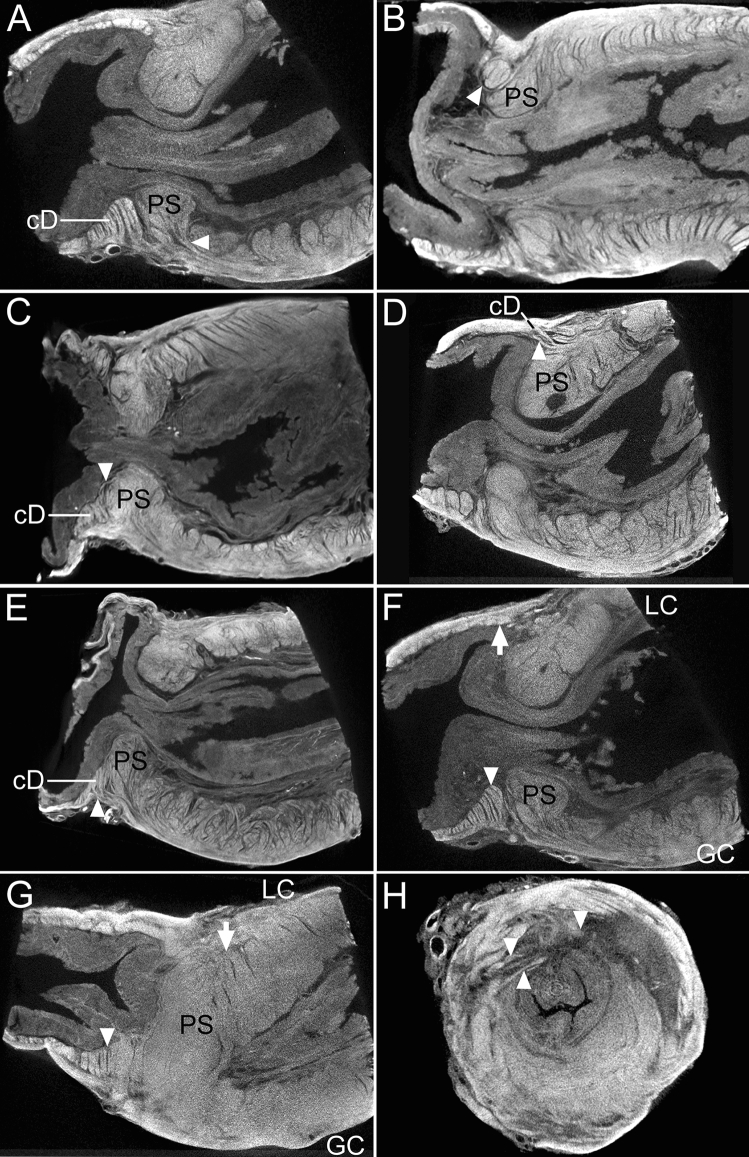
Microcomputed tomography images of the pyloric sphincter (PS). (A) Some outer longitudinal fibers (arrowhead) entered the PS in the longitudinal plane. (B) Some longitudinal fibers (arrowhead) entering the PS were connected to the aboral circular PS bundles in the longitudinal plane. (C) Some inner longitudinal bundles (arrowhead) were divided at the internal surface of the intermediate PS wall to connect to the circular bundles of the duodenum (cD) (arrowhead), adjacent to the PS in the longitudinal plane. (D) Some inner longitudinal bundles (arrowhead) that divided at the internal surface of the aboral end of the PS wall were connected to the cD adjacent to the PS in the longitudinal plane. (E) Some outer longitudinal bundles (arrowhead) were connected to the cD adjacent to the PS. (F) The cD (arrowhead) adjacent to the PS on the greater curvature (GC) side were thicker than those (arrow) on the lesser curvature (LC) side in the longitudinal plane. (G) In the longitudinal plane, the thick PS layer (arrow) on the LC side, and the thick duodenum muscular layer (arrowhead) on the GC side were successively arranged, crossing the gastroduodenal junction in the longitudinal plane. (H) In the axial plane, some muscles bundles (arrowheads) between the PS and duodenum were arranged irregularly.
